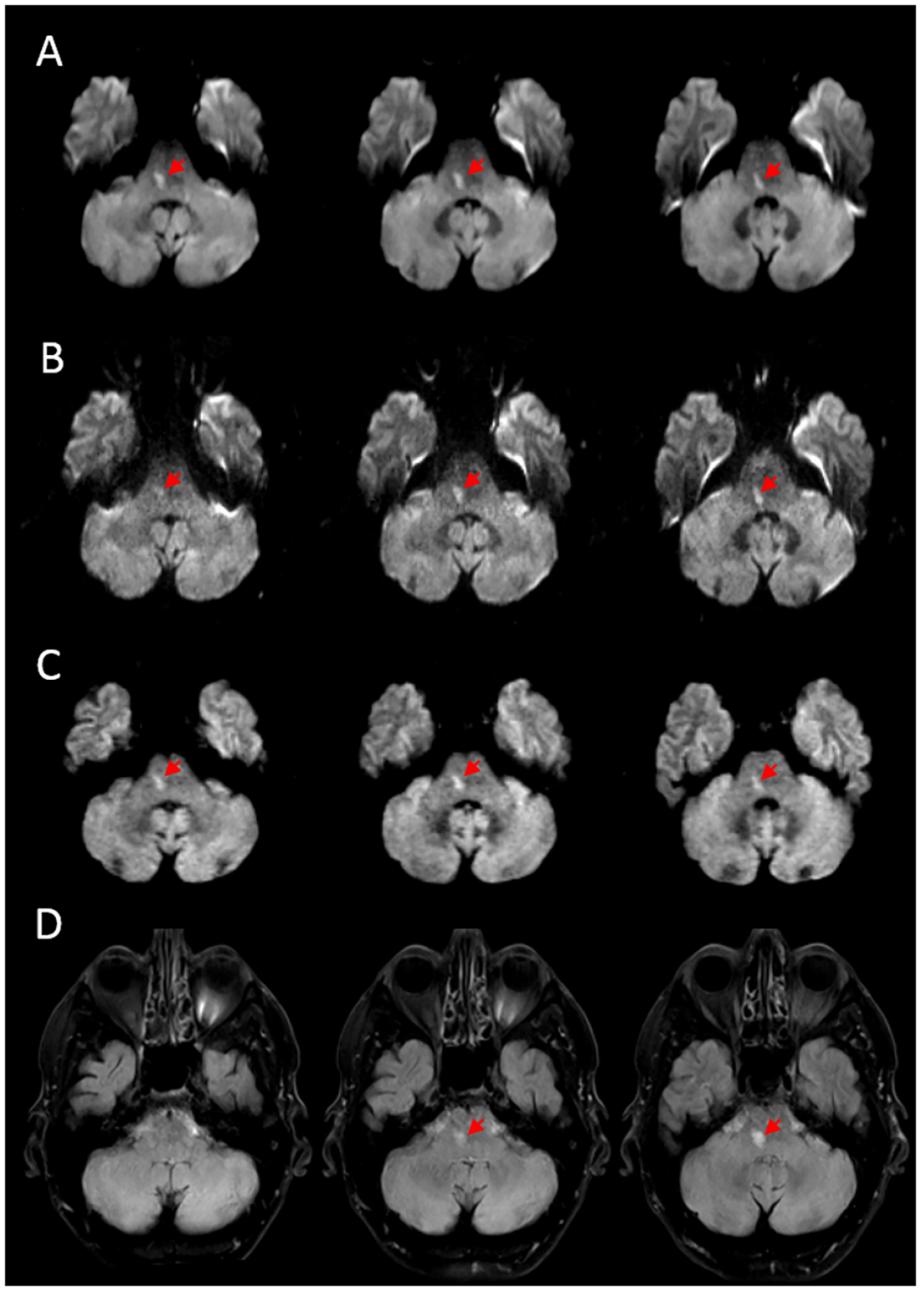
Representative example of agreement between DWI sequences for a patient studied 8 hours after onset of stroke symptoms. Both low- (A) and high-resolution (B) EPI-DWI show a hyperintensity in the right pons spanning three slices. C) STEAM-DWI also shows a hyperintensity in the right pons. D) FLAIR performed five days after baseline scans confirmed the presence of an infarct in the same location. Ischemic lesions are indicated by red arrows.
